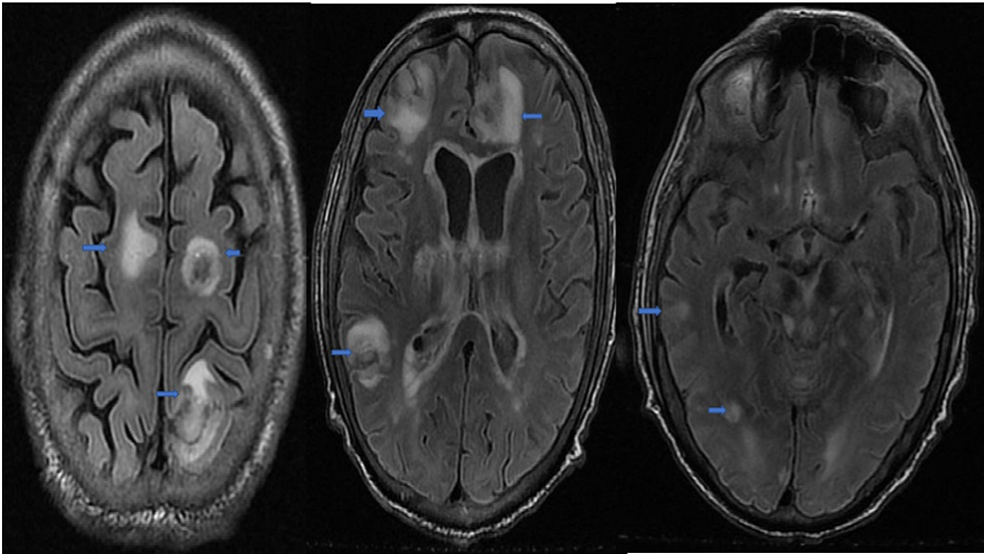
MRI head without contrast Multiple masses with hemorrhage (blue arrows)
